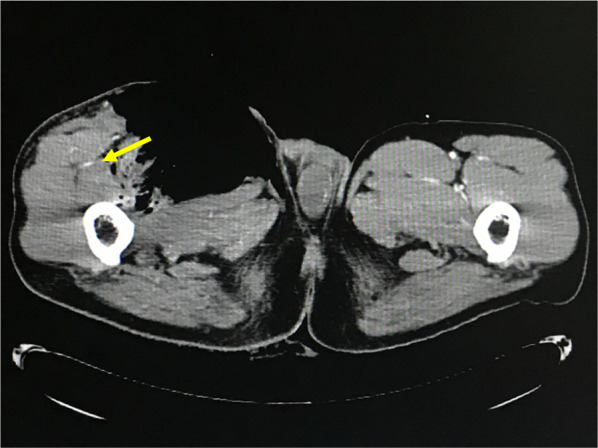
Contrast-enhanced computed tomography image on admission. The right superficial and deep femoral artery were interrupted. However, lateral femoral circumflex artery branches from deep femoral artery seemed patent (yellow arrow). Further examination of distal vessels could not be done because of the need to prioritize resuscitation
